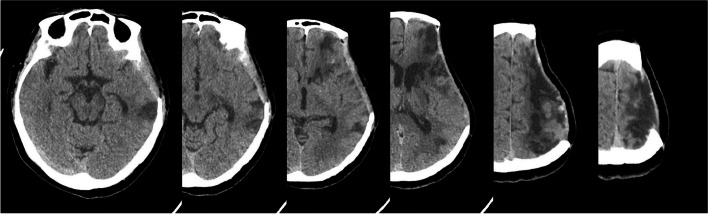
Cranial CT performed 53 days after the operation
